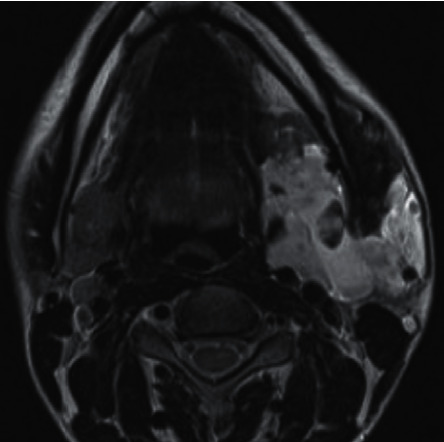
Axial-section *T*2-weighted MRI sequence showing a well-marginated lesion involving whole of the left parotid gland, hyperintense with lobulated hypointense foci within it.
